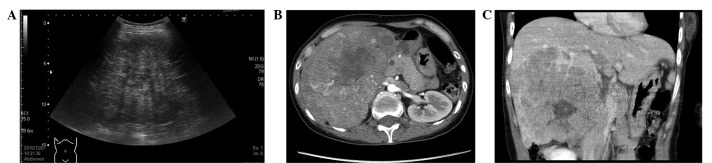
(A) Abdominal ultrasound images revealing a large (>12 cm) mixed echoic hepatic tumor, with ill-defined margins. Computed tomography scans revealing (B) a large hepatic adenoma, with multiple daughter nodules, and (C) multiple daughter nodules in the right lobe of the liver on the coronal view.
